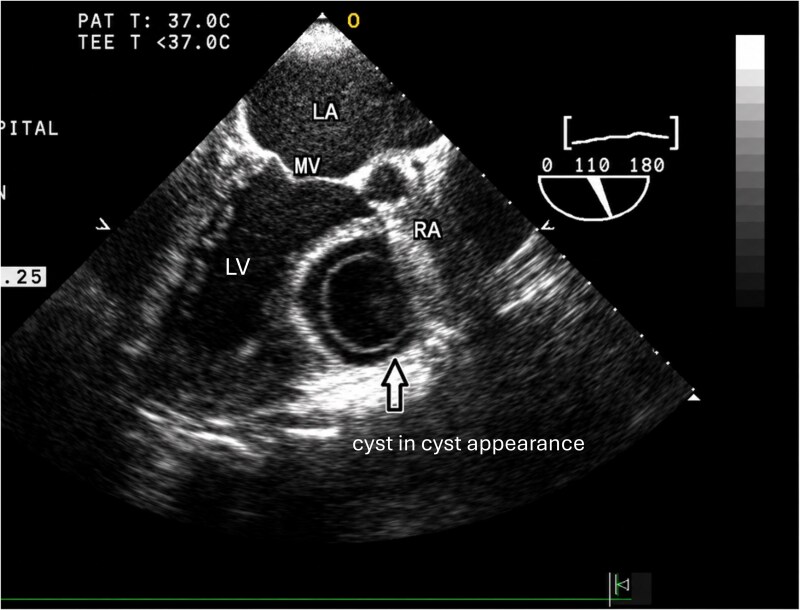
Transoesophageal echocardiography, mid-oesophageal view, demonstrating a well-defined intramyocardial cystic lesion within the interventricular septum (arrow) with a characteristic ‘cyst-in-cyst’ appearance, protruding towards the right ventricular cavity consistent with cardiac hydatid disease.
